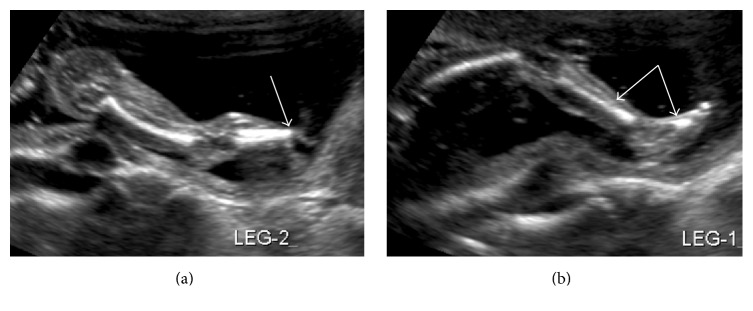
Official ultrasound on 1/13/2016: one lower extremity (left leg, leg 2 in (a)) is incomplete, the tibia fibula terminates abruptly and a foot is not seen. The other lower extremity (right leg, leg 1 in (b)) shows appropriate length for femur, tibia fibula, and a well formed foot. Noted during the examination are floating bands strongly suggestive of amniotic bands.
